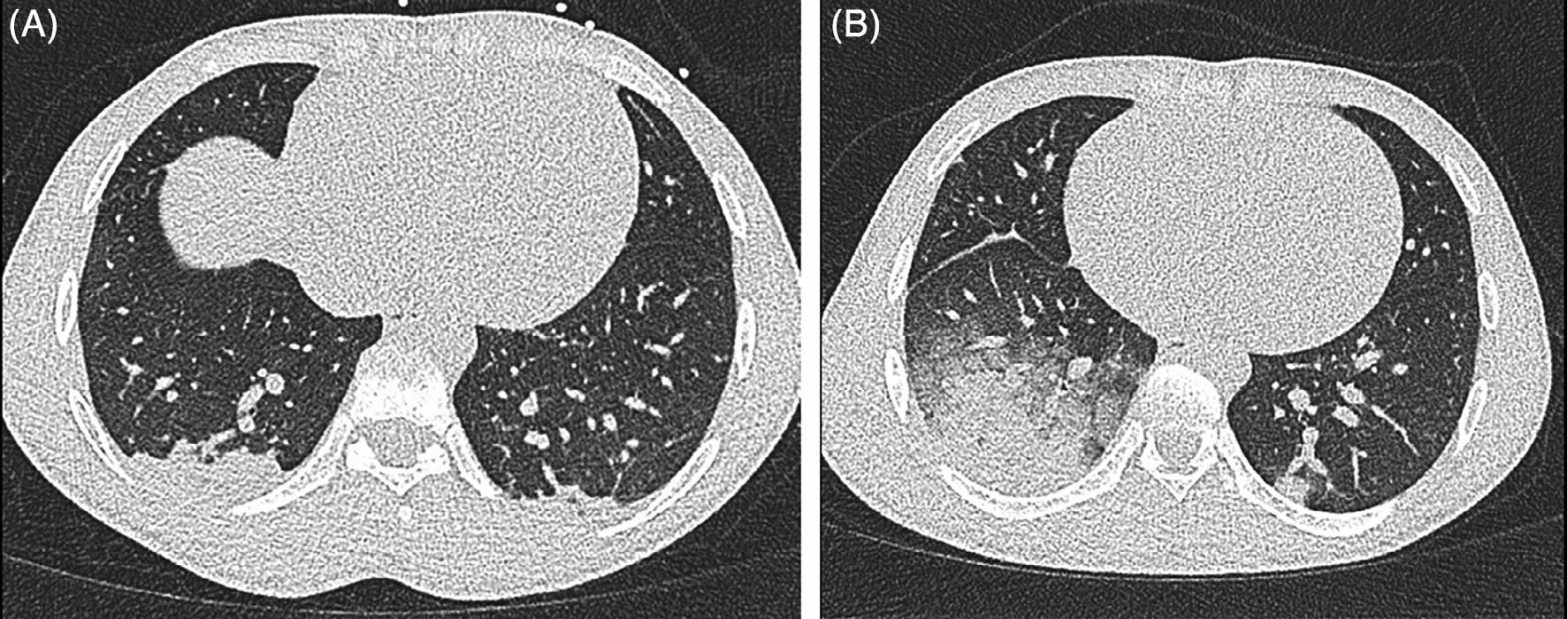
A, Chest CT imaging of patient 1 at first presentation to the emergency room (ER) showing infiltrates at basal fields. B, CT imaging at second ER presentation of patient 1, showing an increase in double‐sided infiltrates
